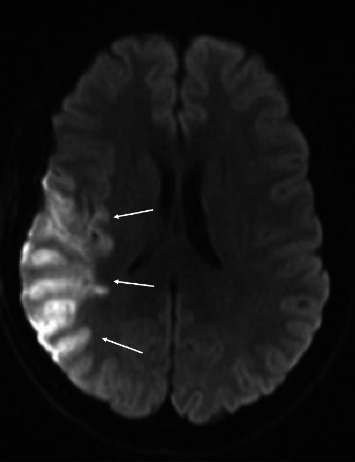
MRI head, diffusion-weighted imaging, demonstrating diffusion restriction along the right MCA territory (denoted by arrows).
